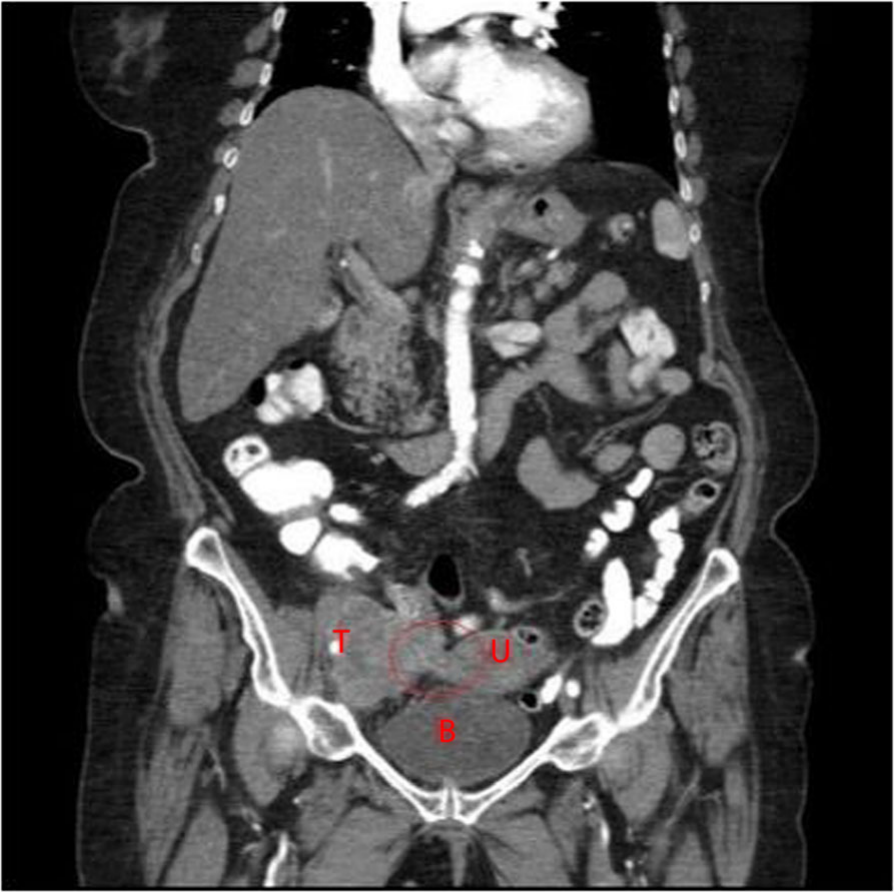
Coronal abdominal computed tomographic scan. *B* Bladder, *T* Tumor, *U* Uterus. Retrospectively, a tissue connection between retroperitoneal tumor and cervix could be recognized
